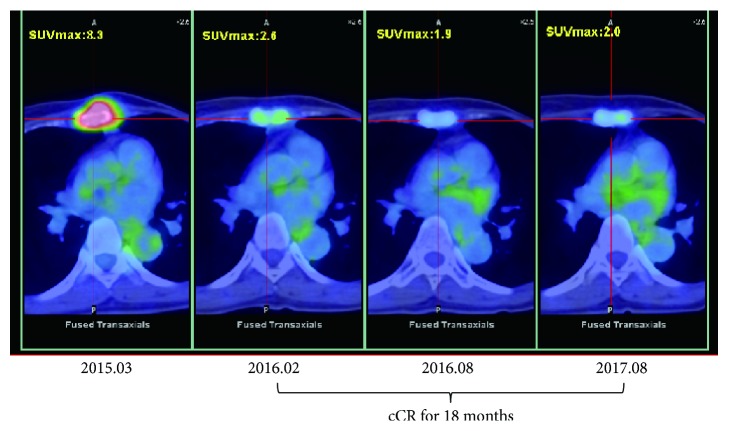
PET-CT of the sternum of Case #17 (assigned as cCR Case 2). The cCR status has been maintained for 18 months to date.
